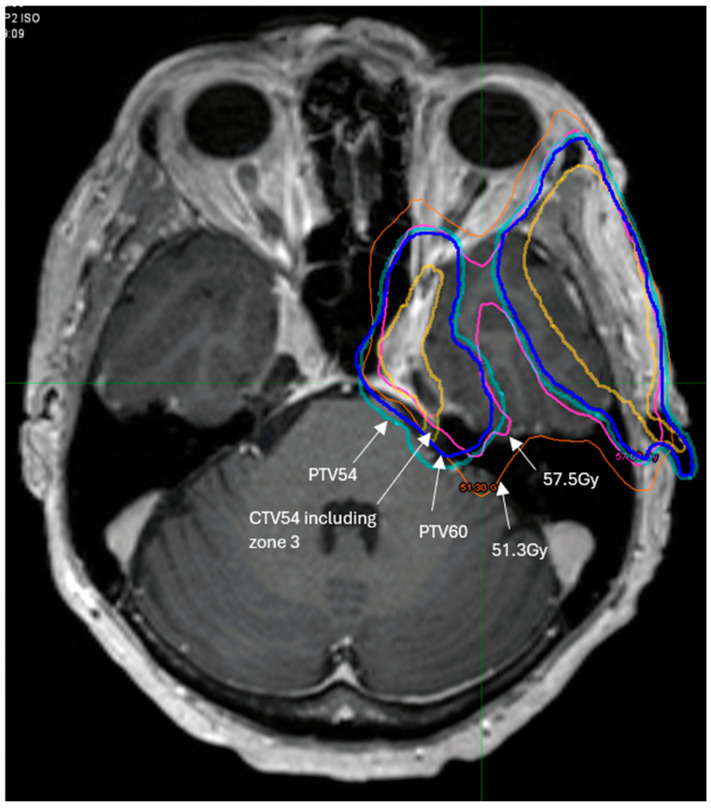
A patient with left V2 zone 2b disease resected with clear margin. Preoperative magnetic resonance neurogram fused with planning CT. PTV 60 (dark blue) encompassed the resected peripheral perineural disease inclusive of the cutaneous primary site on the left cheek (flap repaired) and V2 nerve tract to and including the Trigeminal ganglion. The PTV54 (cyan) extended to the cisternal segment of the left Trigeminal nerve (zone 3). Note the 95% isodose line for PV60 = 57.5 Gy (pink) was prioritised for brainstem constraint (Dmax limit ≤ 54 Gy) over coverage of PTV.
